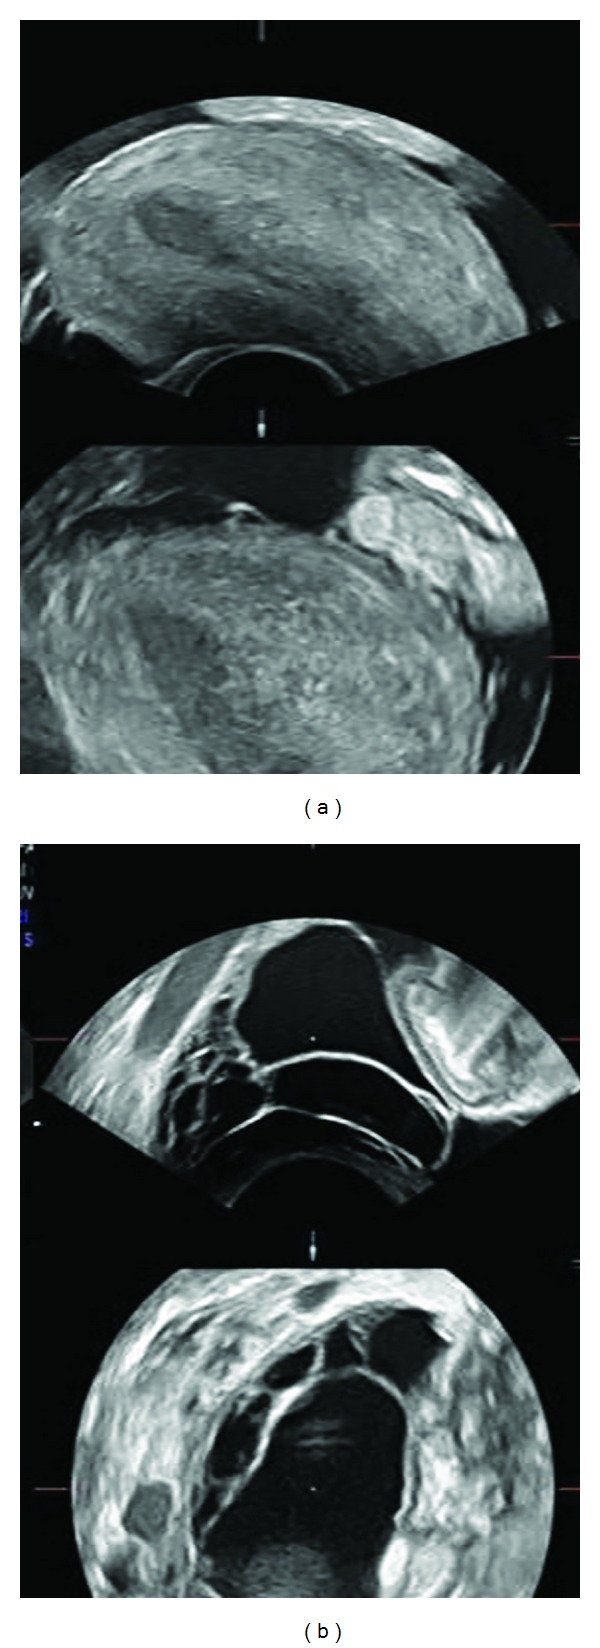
Transvaginal ultrasonography: (a) uterus and ascites; (b) hydrosalpinx.
